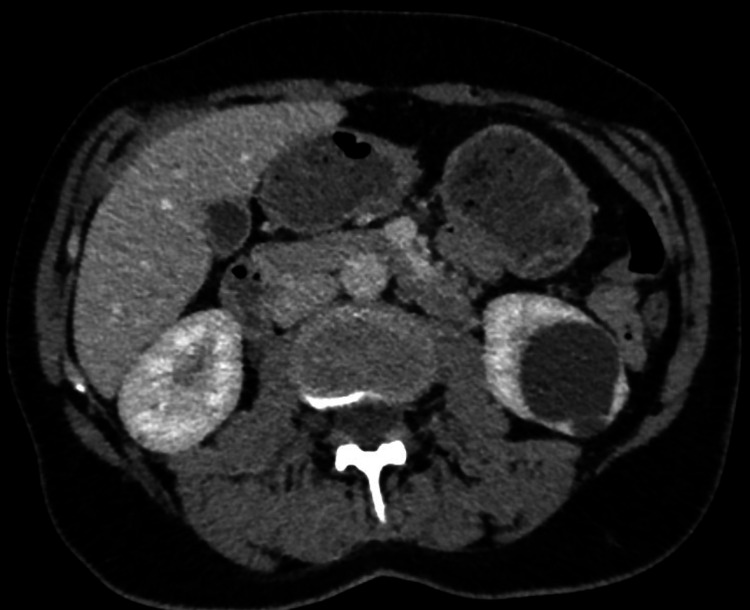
An axial cross-sectional enhanced abdominal computed tomography (CT) scan in a patient genetically proven to have BHD syndrome, showing the presence of a large left non-enhancing simple renal cyst measuring 3.5 cm x 5 cm.
